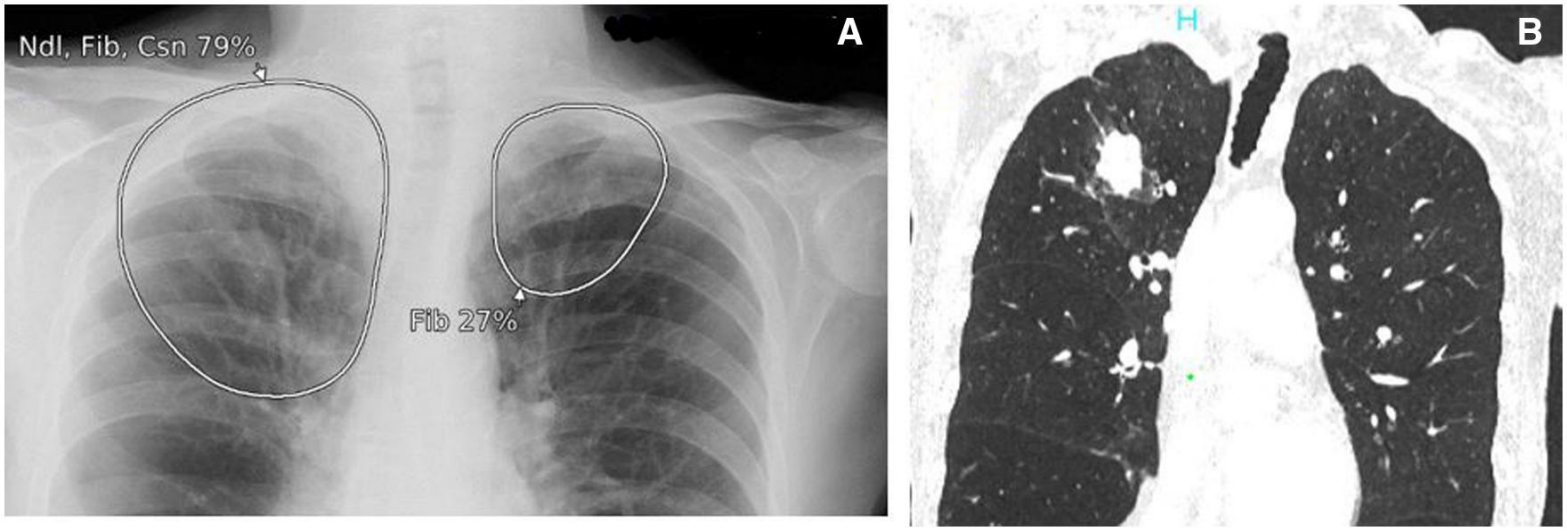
A 85-year-old male with a history of smoking and weight loss underwent a CXR (A) reported as NAD. The AI software demonstrates suspicion of a nodule in the right lung apex. One year and 2 months later, a subsequent CT scan was performed (B) due to the continuation of the same symptoms, demonstrating a right upper lobe mass, later confirmed as stage 3a LC. AI = artificial intelligence; CXR = chest radiograph; LC = lung cancer; NAD = no abnormility detected.
